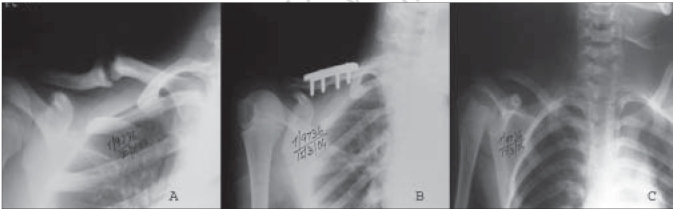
X-ray of shoulder antero- posterior view of an 8 years old female child shows. A) 1½ years old non-union fracture right clavicle. B) Post operative radiograph. C) Radiograph after removal of plate
